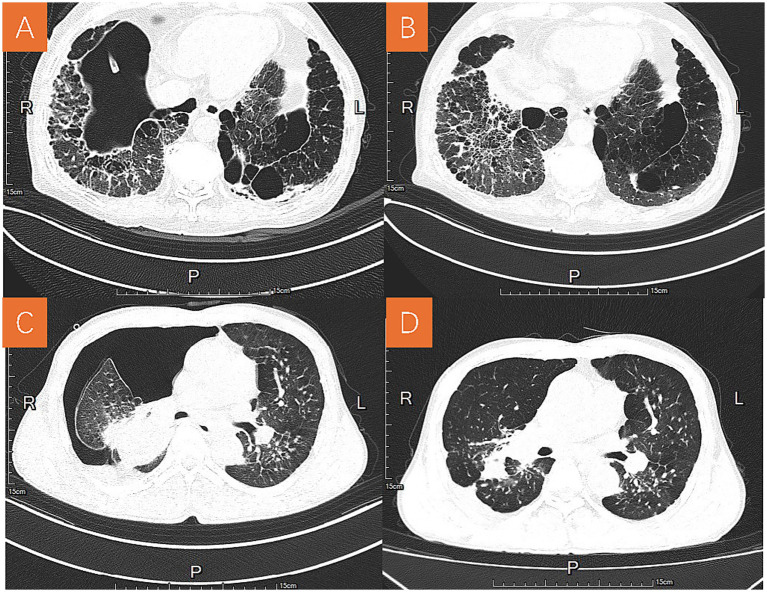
Representative chest CT images before and after treatment in two patients with pneumoconiosis-associated pneumothorax. (A) Chest CT images of patient A before treatment. (B) Chest CT images of patient A after treatment. (C) Chest CT images of patient B before treatment. (D) Chest CT images of patient B after treatment, showing improvement of pneumothorax and lung re-expansion.
